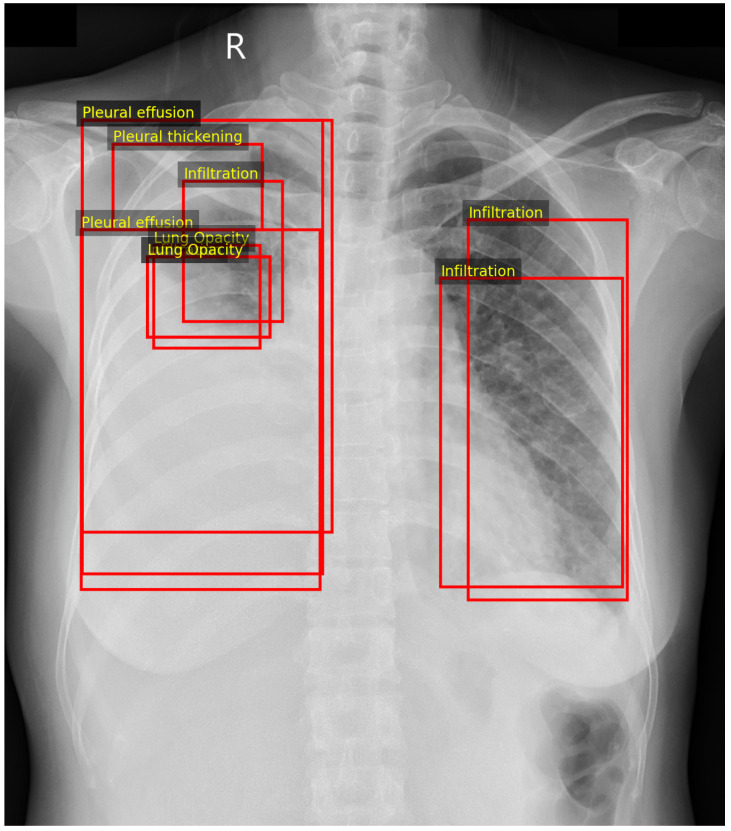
Radiologist bounding-box annotations for the selected multi-label VinBigData study. Red frames denote expert-annotated regions of interest, and the letter “R” indicates the right side of the chest radiograph. Overlapping boxes indicate pleural effusion, pleural thickening, lung opacity, and infiltration, reflecting the coexistence of multiple abnormalities within the same examination.
